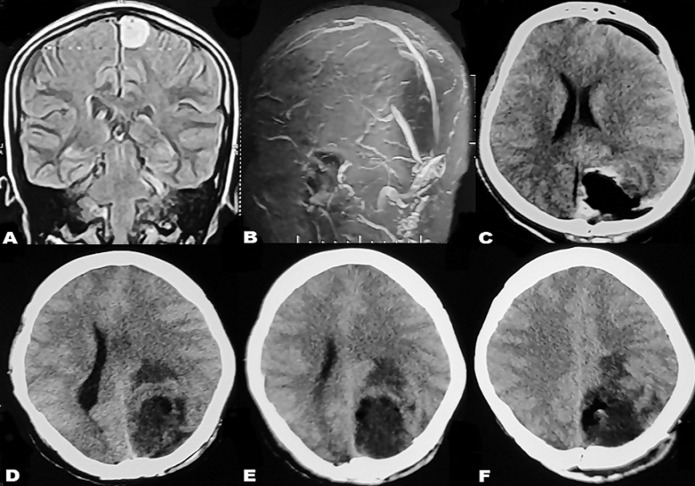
(A) contrast-enhanced MRI brain showing left posterior parasagittal meningioma; (B) grossly normal preoperative MR venogram of the patient; (C) contrast-enhanced CT brain showing gross total tumor excision; (D,E,F) CT brain on 15^th^ post-operative day: extensive cerebral edema with acute parieto-occipital infarct
